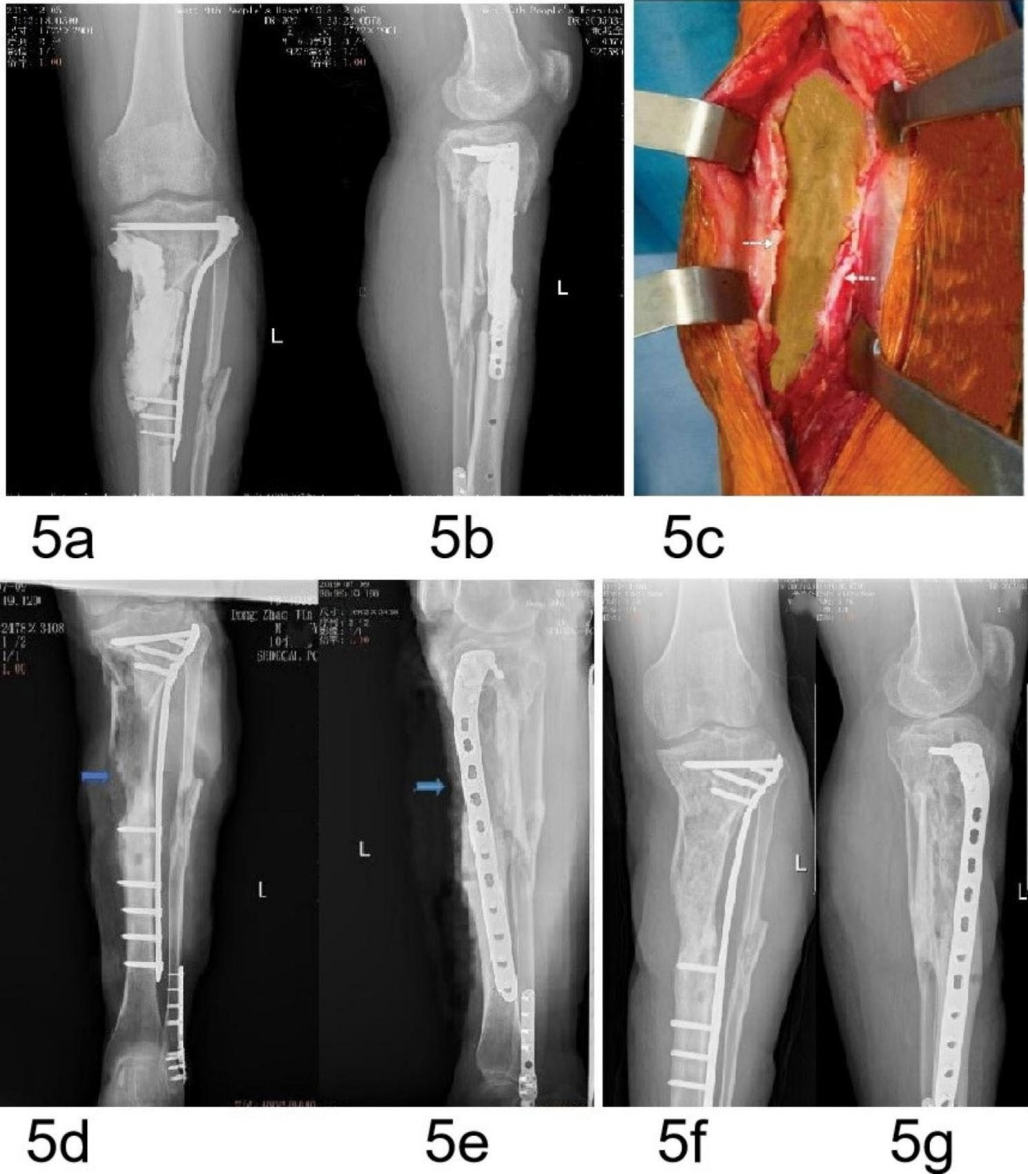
A 37-year-old male patient with a grade I IMSO. (a,b) AP and lateral radiographs showing that the cement spacer of the PMMA filled in the defects after debridement for infection of upper open tibial fractures. (c) Intraoperative exploration revealing thickening (2–3 mm) and hardening of the IM (white arrow). (d,e) AP and lateral radiographs showing indistinct new bone formation at nine months after insertion of the PMMA. (f,g) AP and lateral radiographs showing clinical healing of the fractures and defects after bone grafting
